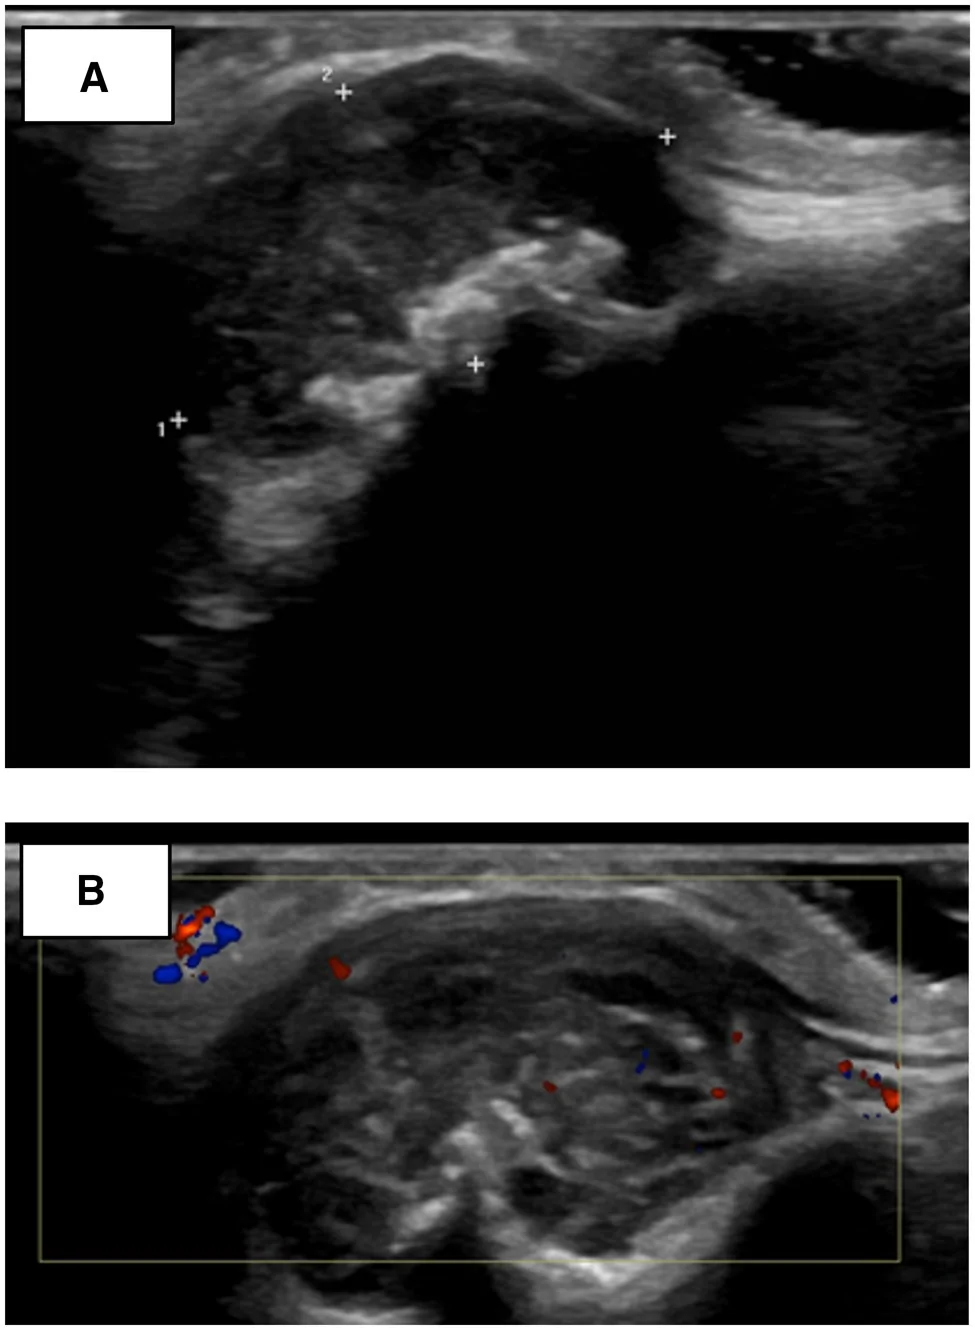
(A) High-resolution transverse ultrasound of the neck. Ultrasound demonstrates a 23 × 12 mm well-defined lesion with heterogeneous echogenicity at the level of the hyoid bone, containing internal calcific foci. The lesion appears closely related to the underlying cartilaginous structures and is separate from the thyroid gland. (B) Colour Doppler ultrasound of the neck. Colour Doppler ultrasound demonstrates minimal internal vascularity within the lesion, which measures approximately 23 × 12 mm and exhibits heterogeneous echogenicity with internal calcific foci at the level of the hyoid bone.
